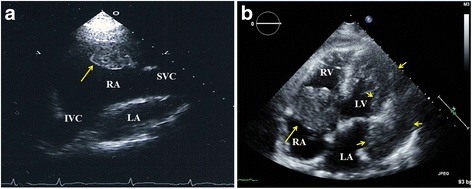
Representative cases incorrectly diagnosed by transthoracic echocardiography. a. Horizontal section of a right parasternal view. Thrombus attached to RA appendage (arrow). We misdiagnosedthis as an RA myxoma. b. Left parasternal four-chamber view. We could not diagnose the tumor type before surgery. A leiomyosarcoma arose from the coronary sinus and was detected around the LV (arrows). RA = right atrium, LA = left atrium, IVC = inferior vena cava, SVC = superior vena cava, LV = left ventricle, RV = right ventricle
